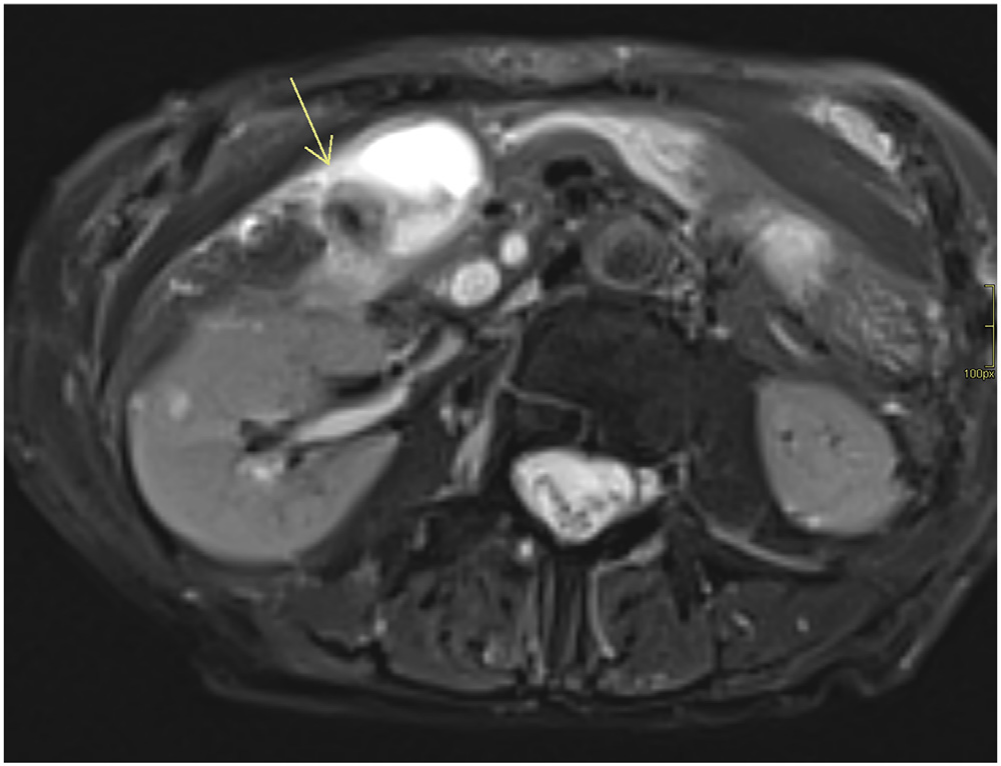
Axial T2-weighted MRCP image showing a distended gallbladder with significant wall edema and a “whirl sign” (arrow) involving the cystic duct and artery, indicative of torsion.
